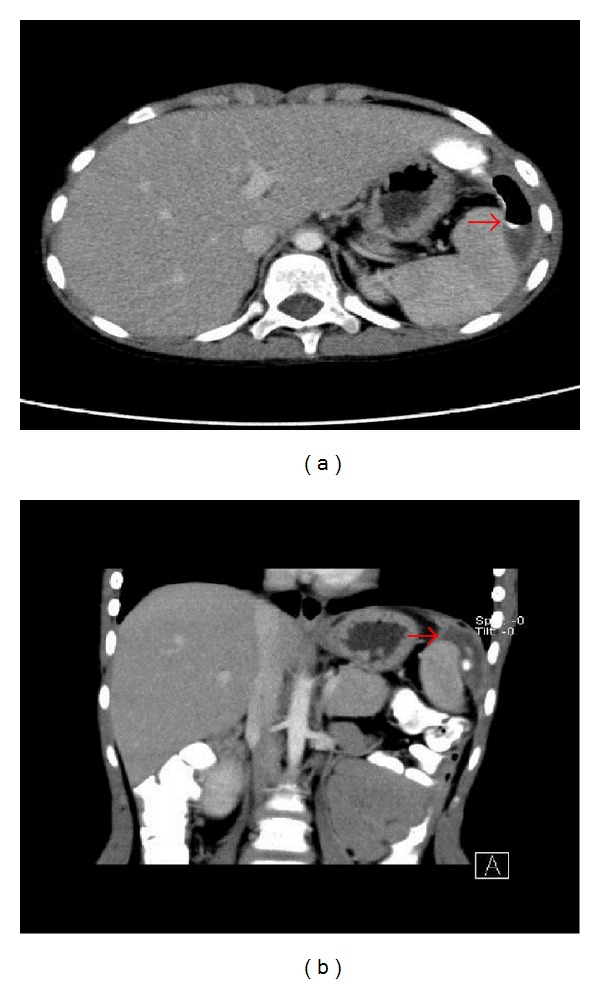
Axial (a) and Coronal (b) abdominal CT projections (with enteric contrast) demonstrate a perisplenic hydroaerial collection with a dense component compatible with enteric content (arrows). A thin fistula (*) connects the collection to the colonic lumen. A further retroperitoneal fluid collection is detectable at the left parietal-colonic region.
